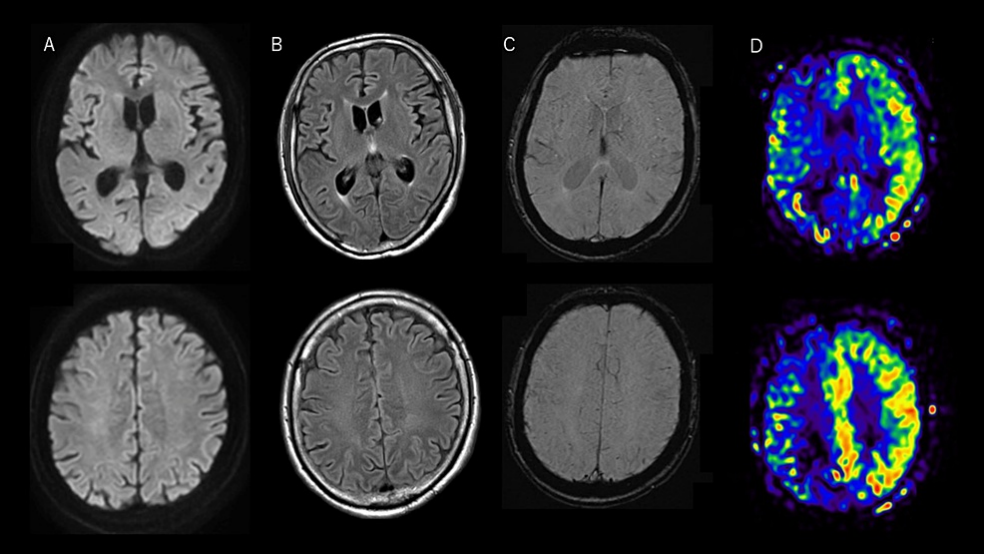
Magnetic resonance imaging after admission (A) Diffusion-weighted imaging (DWI), (B) fluid-attenuated inversion recovery (FLAIR), (C) susceptibility-weighted imaging (SWI), and (D) pulsed continuous arterial spin labeling (pCASL) with post-labeling delay (PLD) of 1,500 ms DWI/FLAIR/SWI did not reveal any abnormalities. However, ASL detected unilateral hemispheric hyperperfusion
